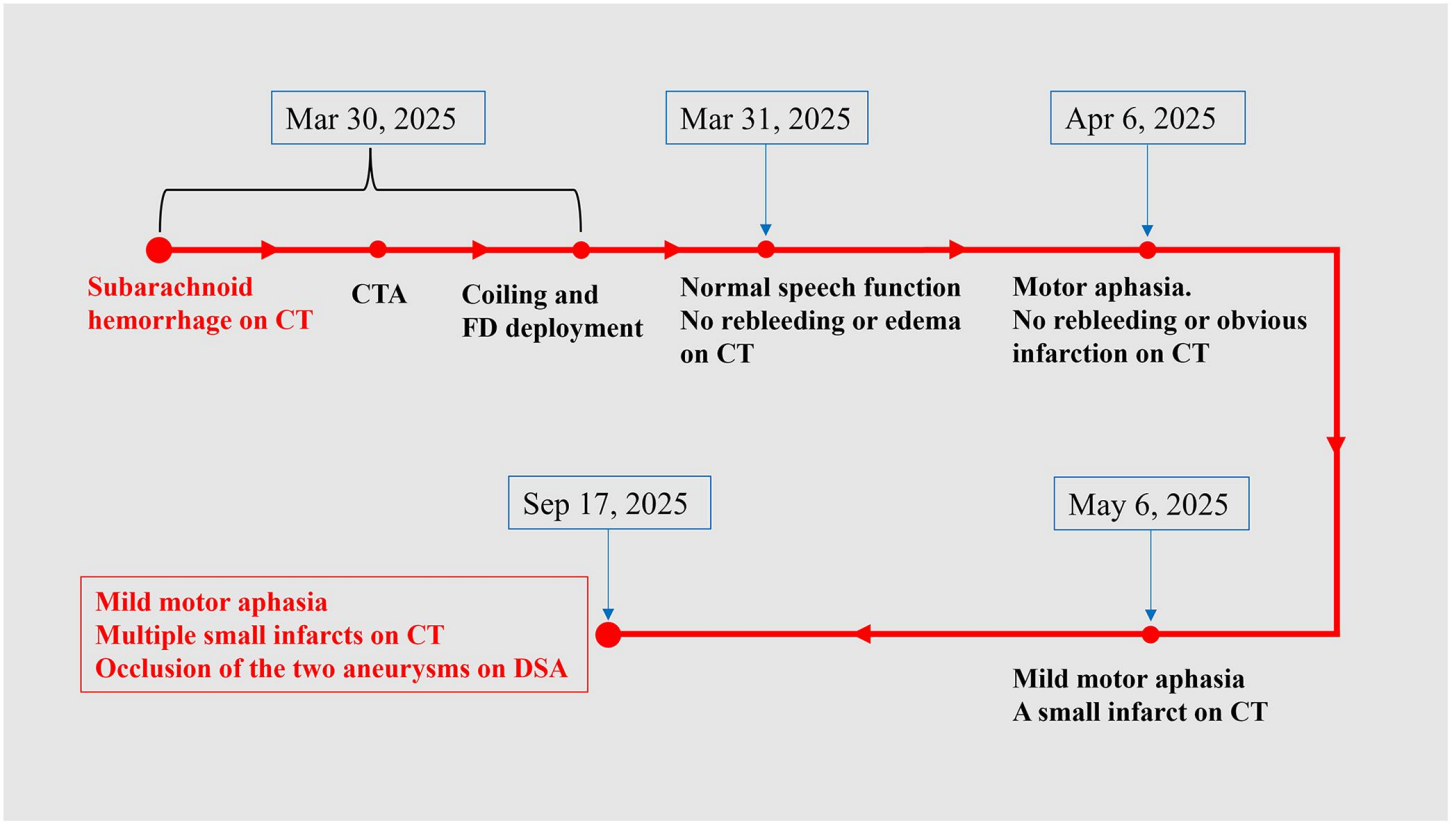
Patient timeline. CT, computed tomography; CTA, computed tomography angiography; DSA, digital subtraction angiography; FD, flow diverter.
